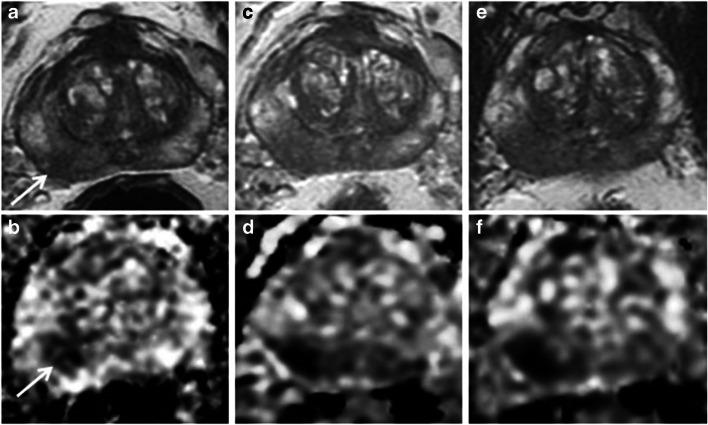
PRECISE score 4. A 66-year-old patient at AS enrolment, with PSA of 3.09 ng/ml. Top row: T2 axial; bottom row: ADC maps. a, b 2013 MRI shows a Likert 5 lesion in the right mid PZ measuring 12 mm × 7 mm (arrows). Initial biopsy showed Gleason 3 + 3 disease. c, d 2015 MRI lesion increased to 15 mm × 9 mm (PRECISE 4). e, f 2017 MRI lesion further increased in size to 17 mm × 10 mm. Targeted biopsy shows Gleason 3 + 4 = 7 up to 6 mm and replacing 85% of both cores. Patient underwent radiotherapy
